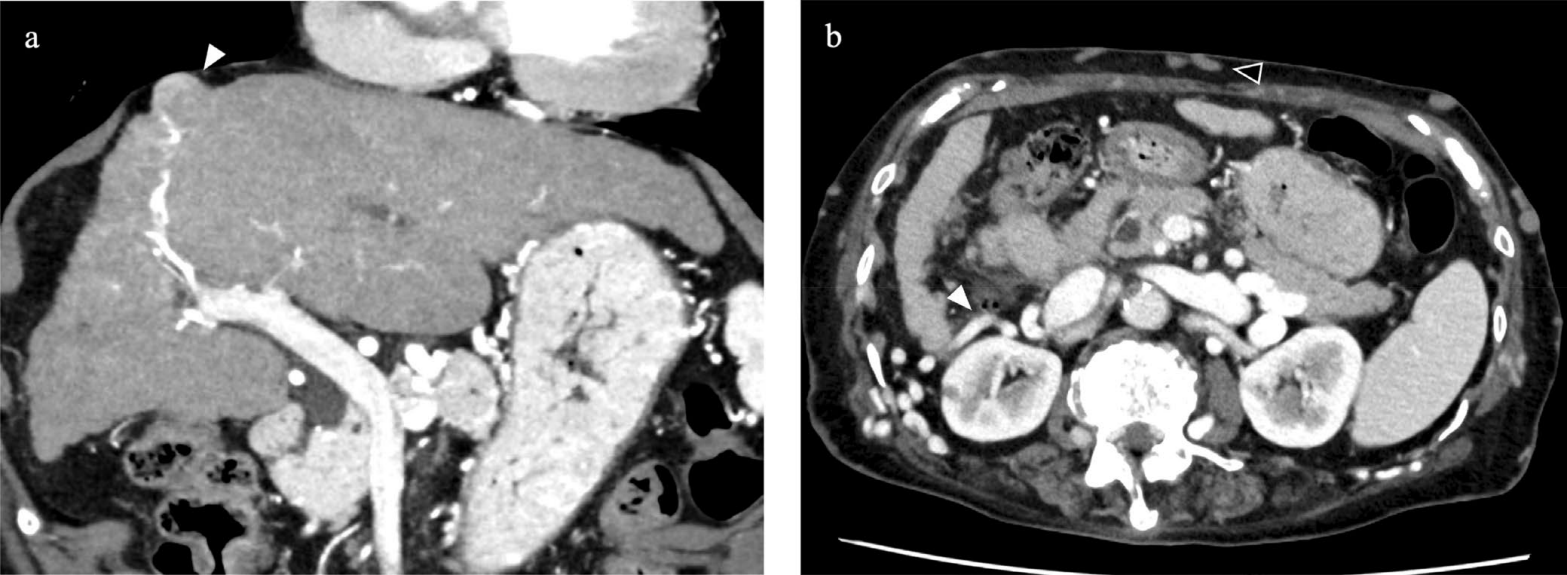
E‐CT examinations revealed a small tumor on the surface of liver segment 8 (white arrowhead). The liver morphology was suggestive of cirrhosis (a). Well‐developed collateral vessels were identified in the retroperitoneum (white arrowhead) and the abdominal wall (black arrowhead) (b).
